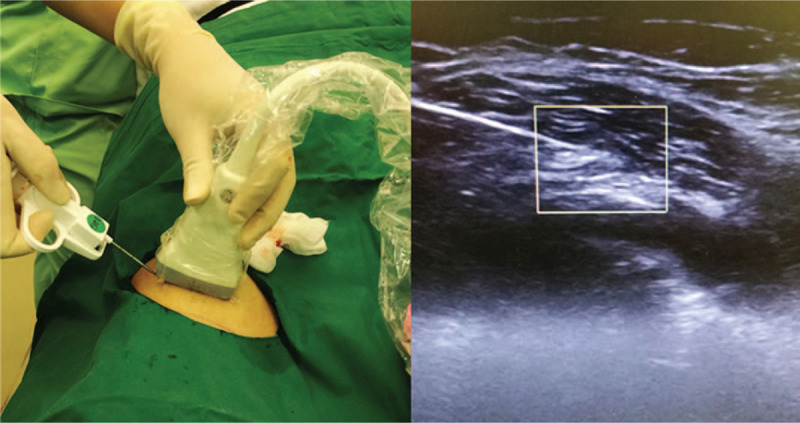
Ultrasound-guided core needle soft tissue biopsy in the leg.
